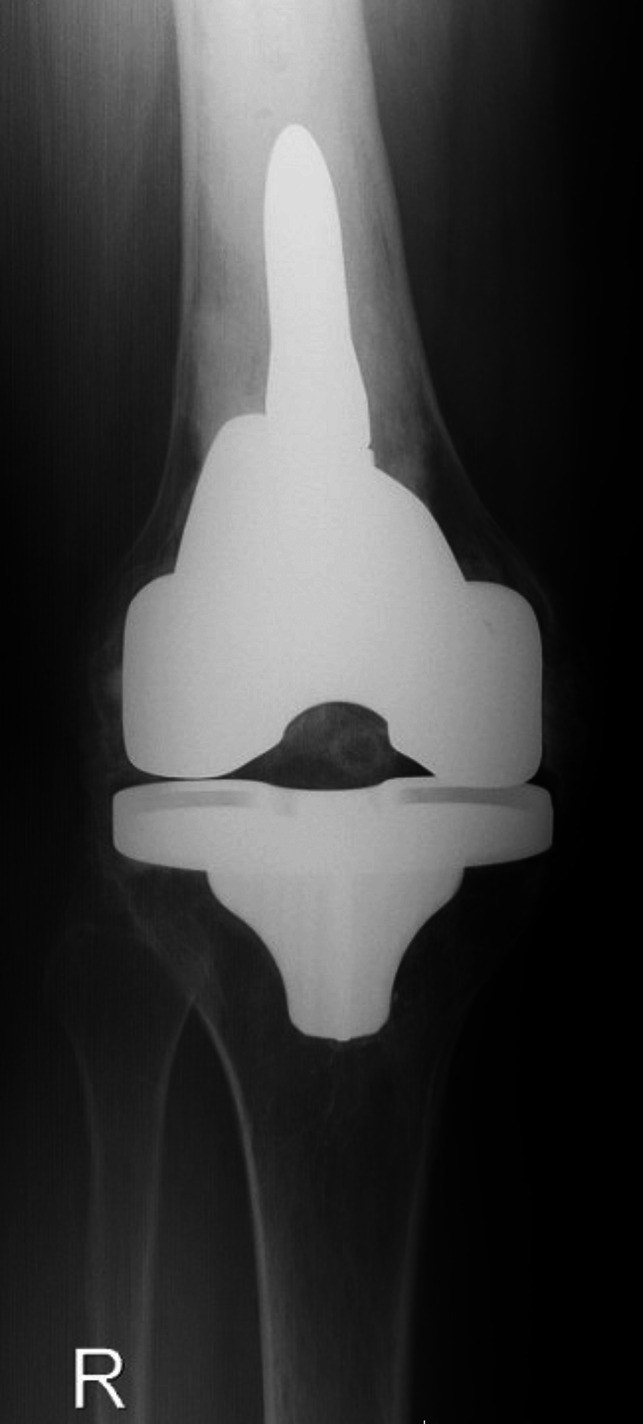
AP radiograph of the right knee.
